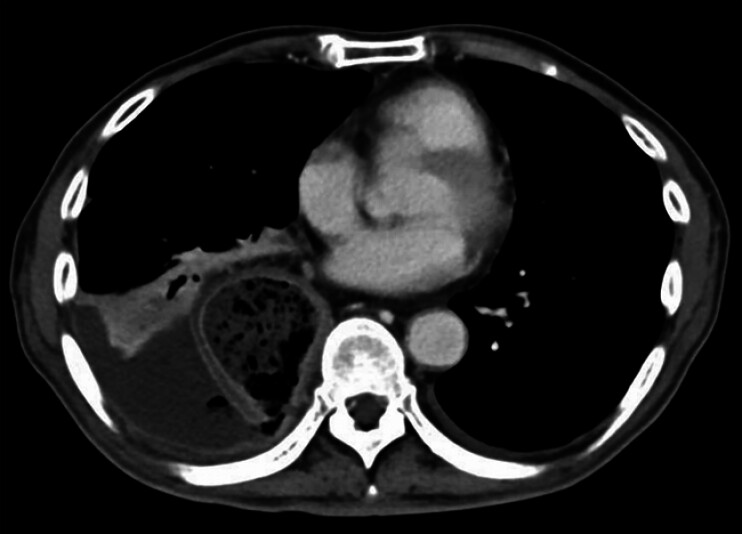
Computed tomography (CT) revealed the presence of gastric tube contents, a reduction in the thickness of the gastric tube wall as well as the presence of pleural effusion in the right posterior lower lung field.
